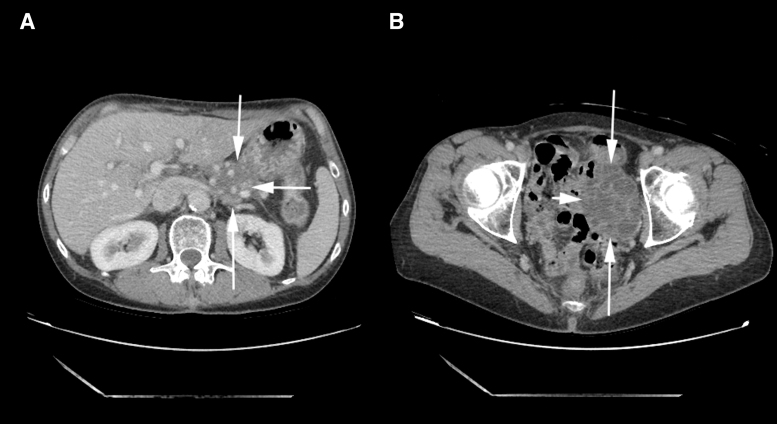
Computed tomography abdomen and pelvis showing the pancreatic and ovarian masses. (A) Mass lesion (5.9 × 3.6 cm) replacing the distal body and tail of the pancreas (*arrows*) with encasement of the adjacent vascular structures. The mass is inseparable from the posterior wall of the stomach and the left adrenal gland. (B) Cystic mass (6.9 × 5.6 cm) in the left adnexal region (*arrows*).
